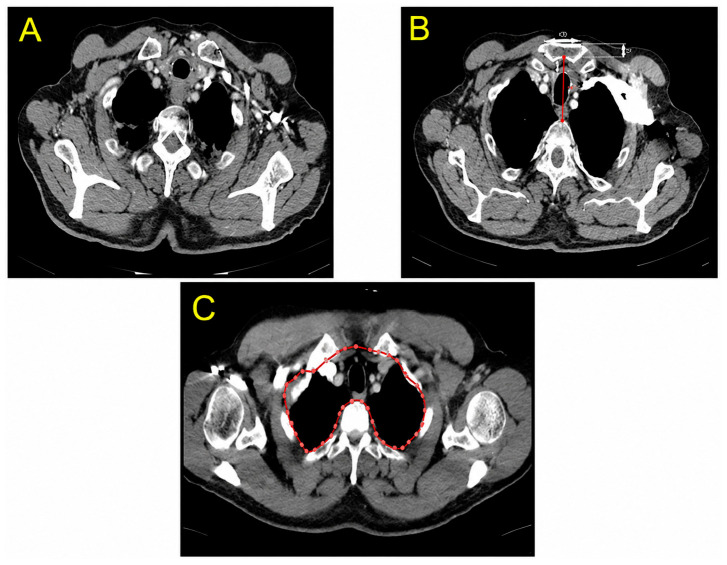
Radiologic measurement of thoracic inlet parameters on preoperative computed tomography images. (A,B) Linear measurements include sterno-tracheal distance (STD), sterno-vertebral distance (SVD), and interclavicular distance (ICD). (C) Thoracic inlet area (TIA) calculation based on predefined linear measurements. All images were obtained from the authors’ institutional database.
